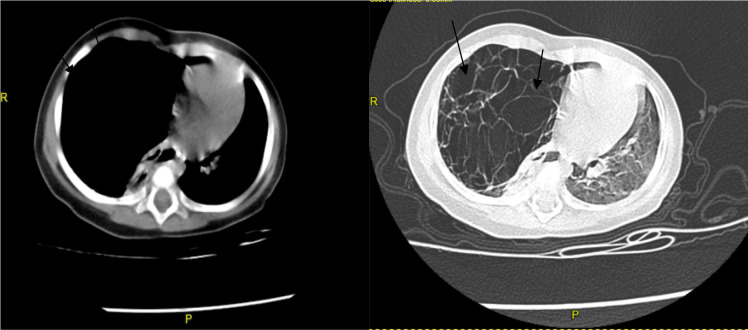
Computed tomography scan an 8-month-old infant with congenital pulmonary malformations.
